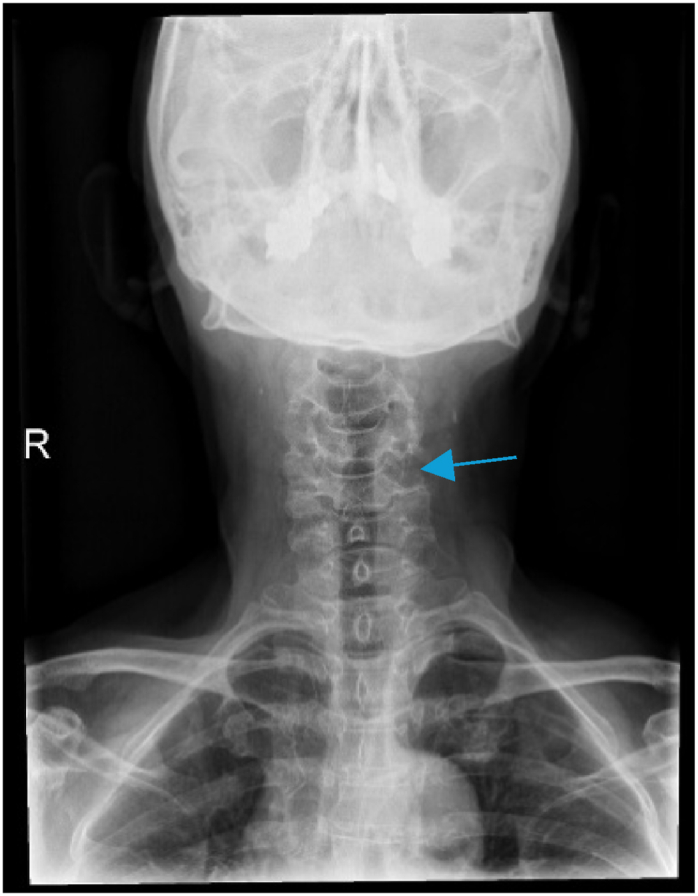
Postoperative X-ray showing the foraminotomy window.
